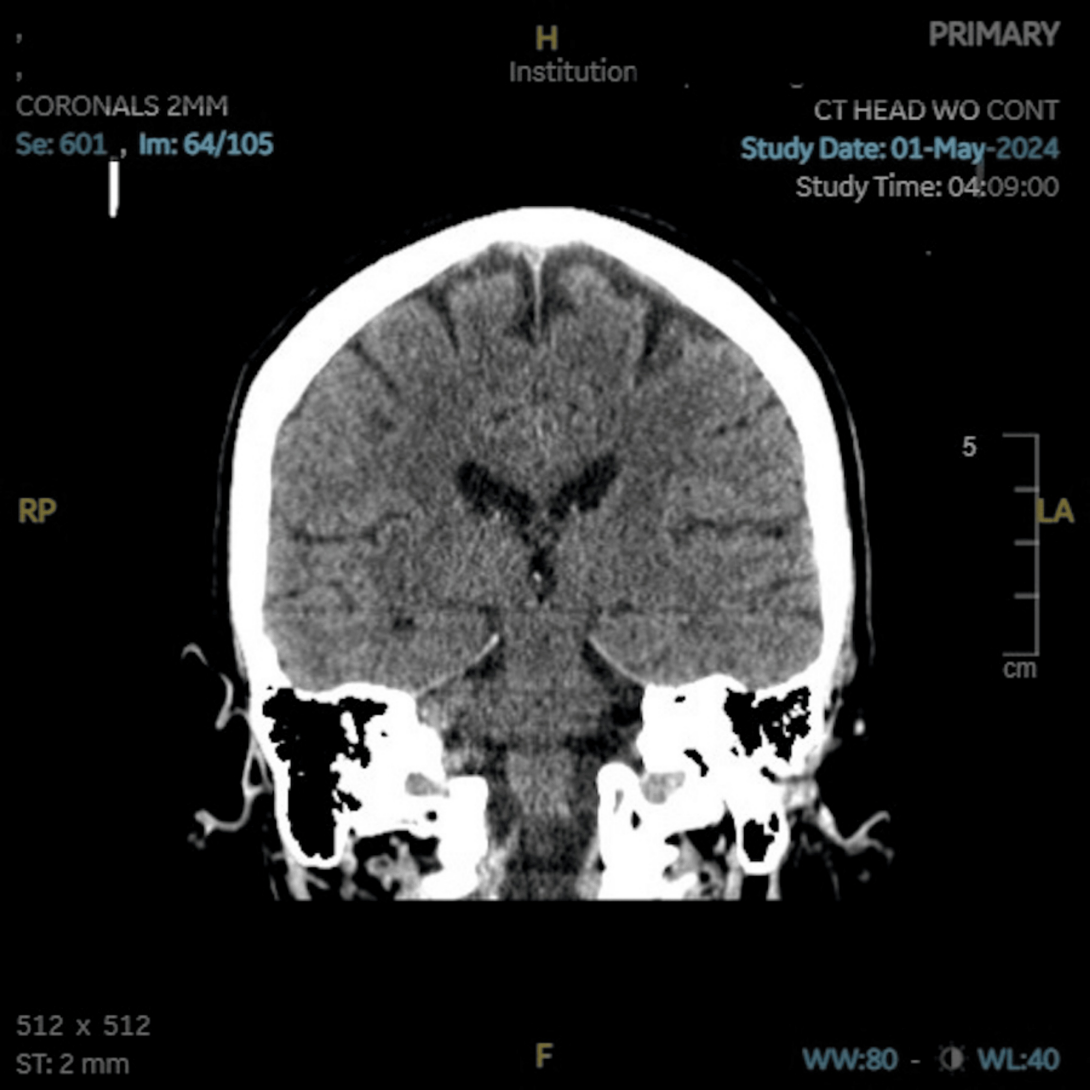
CT of the head with coronal view demonstrating no subdural hematoma
